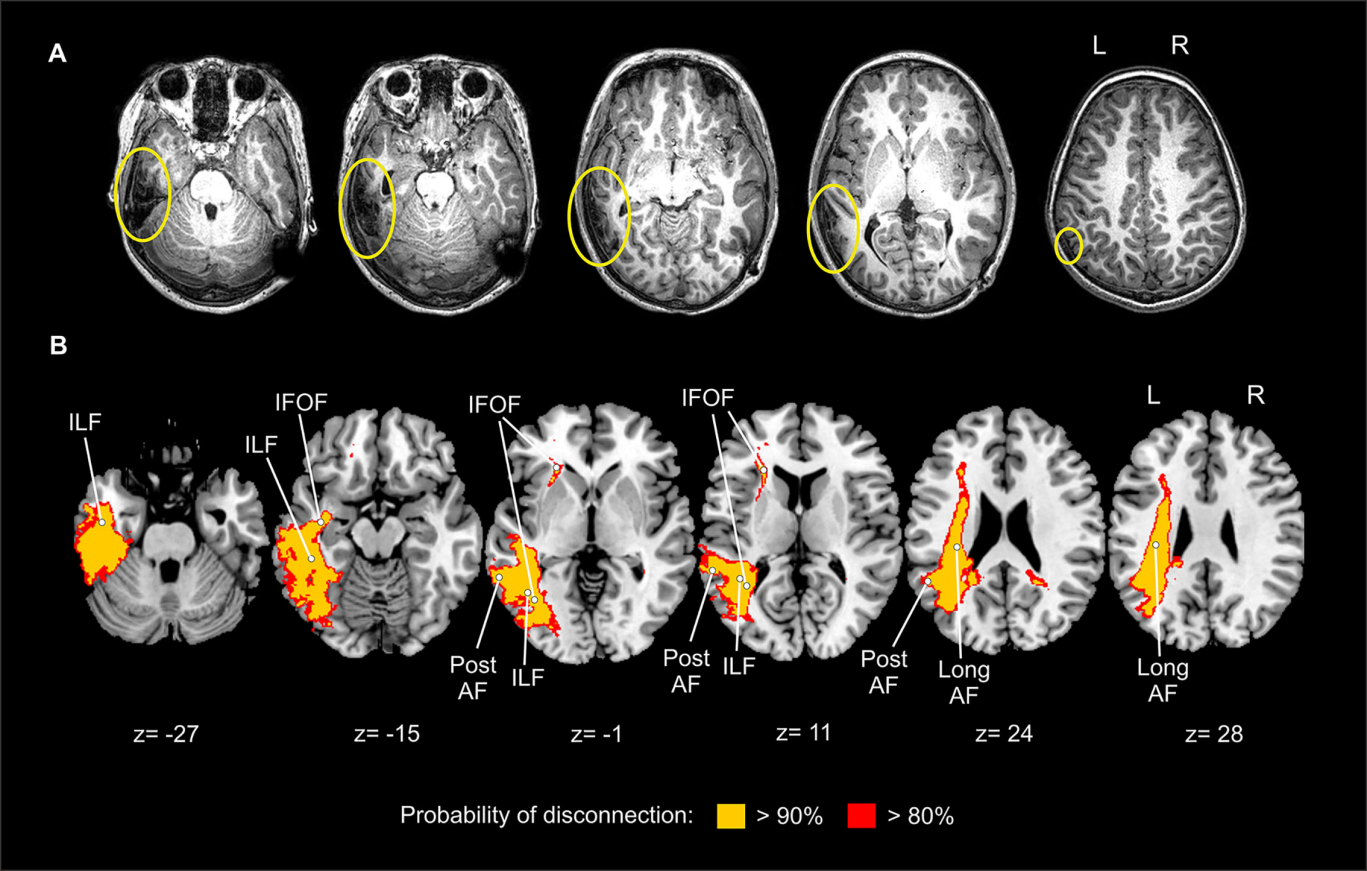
Depiction of the brain lesion and disconnection pattern. (A) MRI T_1_-weighted image showing the lesion in the left temporo-parietal cortex in native space. Yellow circles indicate brain lesions. (B) Probability of disconnection of brain areas not directly affected by the lesion as revealed by the *Disconnectome map* software. Two different probability thresholds are presented. The disconnection map is overimposed on a brain template in standard MNI space. L, left; R, right; ILF, inferior longitudinal fasciculus; IFOF, inferior fronto-occipital fasciculus; post AF, arcuate fasciculus posterior segment; long AF, arcuate fasciculus long segment.
